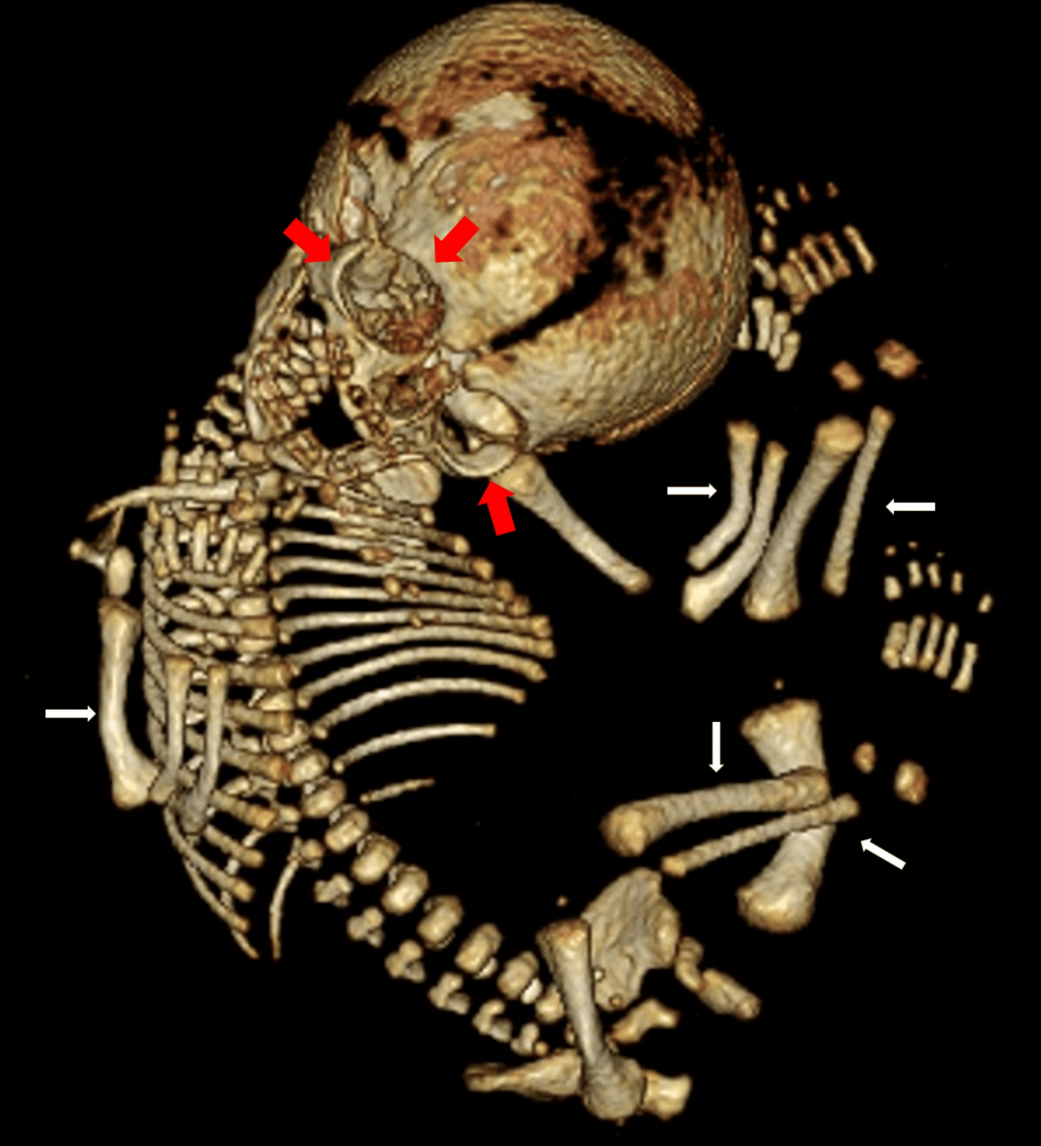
3D CT of the third pregnancy at 35 weeks of gestation The fetal long tubular bones were curved and shortened (white arrow), with mild hypoplasia of the thorax, but the skull base and orbit were not hypoplastic (red arrow). 3D CT: three-dimensional computed tomography
